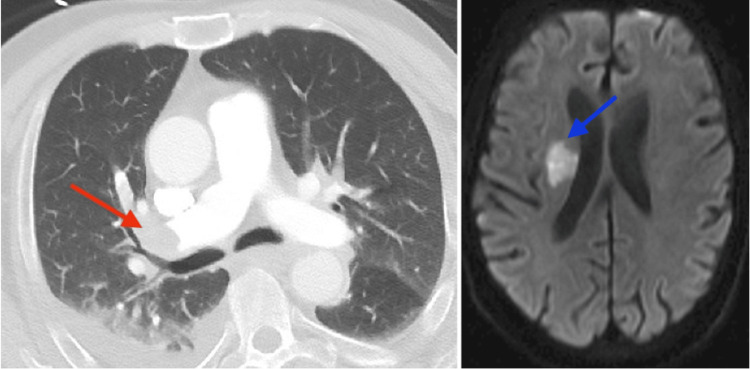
Results of imaging from code stroke *Left*: Computed tomography pulmonary embolus protocol. The embolus is shown by the red arrow. *Right*: Diffusion-weighted magnetic resonance imaging of the brain. Ischemic infarct is shown by the blue arrow.
